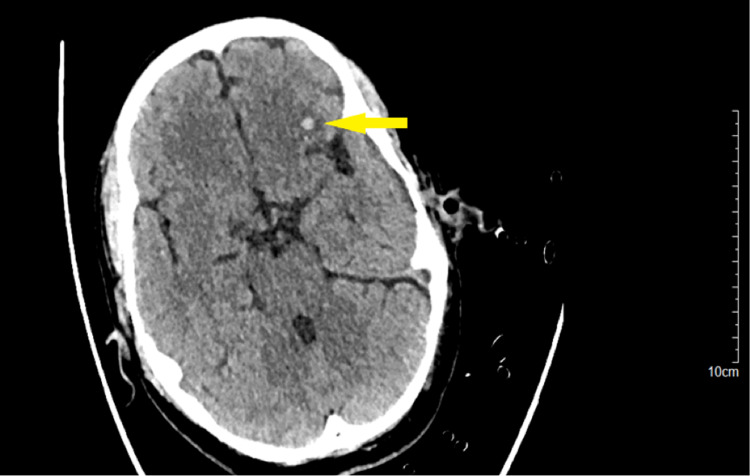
Non-contrast CT scan of the patient showing contusion in the left frontal lobe.
